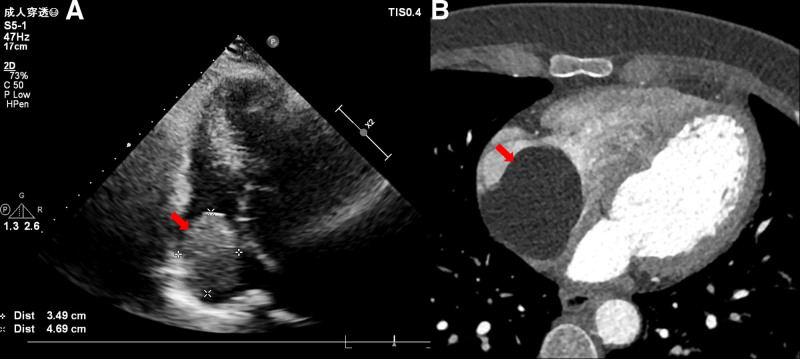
The images show a mass in the right atrium. (A) Echocardiography transcardial apex section: a 4.69 × 3.49 cm^2^ mass in the right atrium, with a regular shape, no lobulation, attached to free wall, and mobile (red arrow). (B) Cardiac CTA perfusion imaging demonstrated a homogenous low–density mass (−114 HU) in the right atrium, measuring 6.0 × 4.1 cm^2^, with a clear boundary. No enhancement was observed on the enhanced scan (red arrow). CTA = computed tomography angiography.
